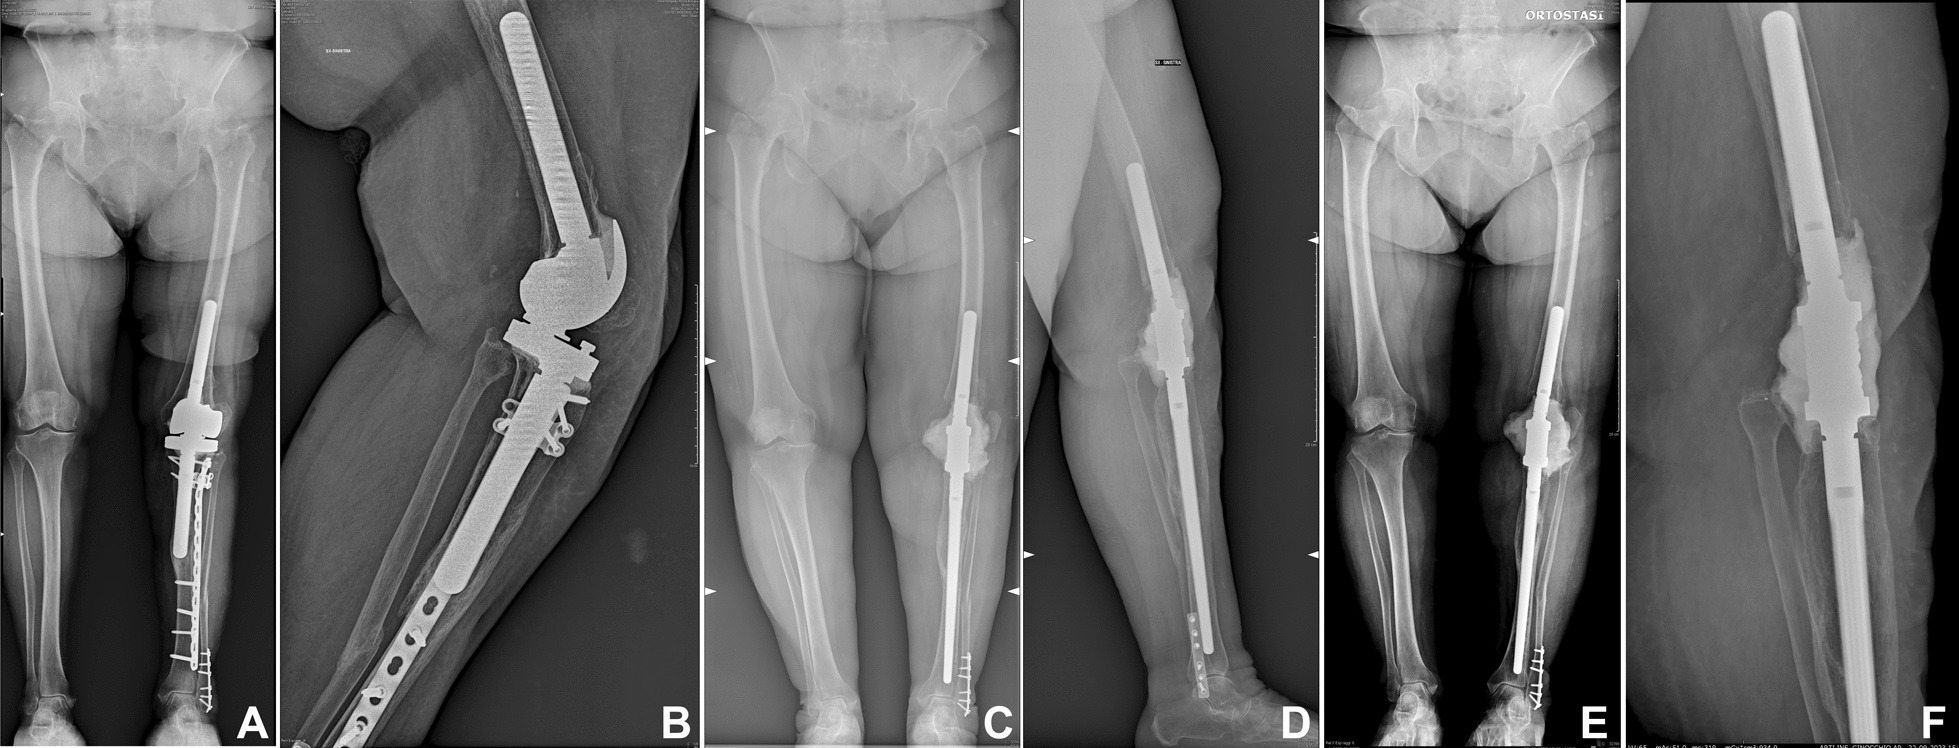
A, B Preoperatory X-rays, C, D X-ray at 21 days post-surgery, E, F 1-year follow up X-rays
